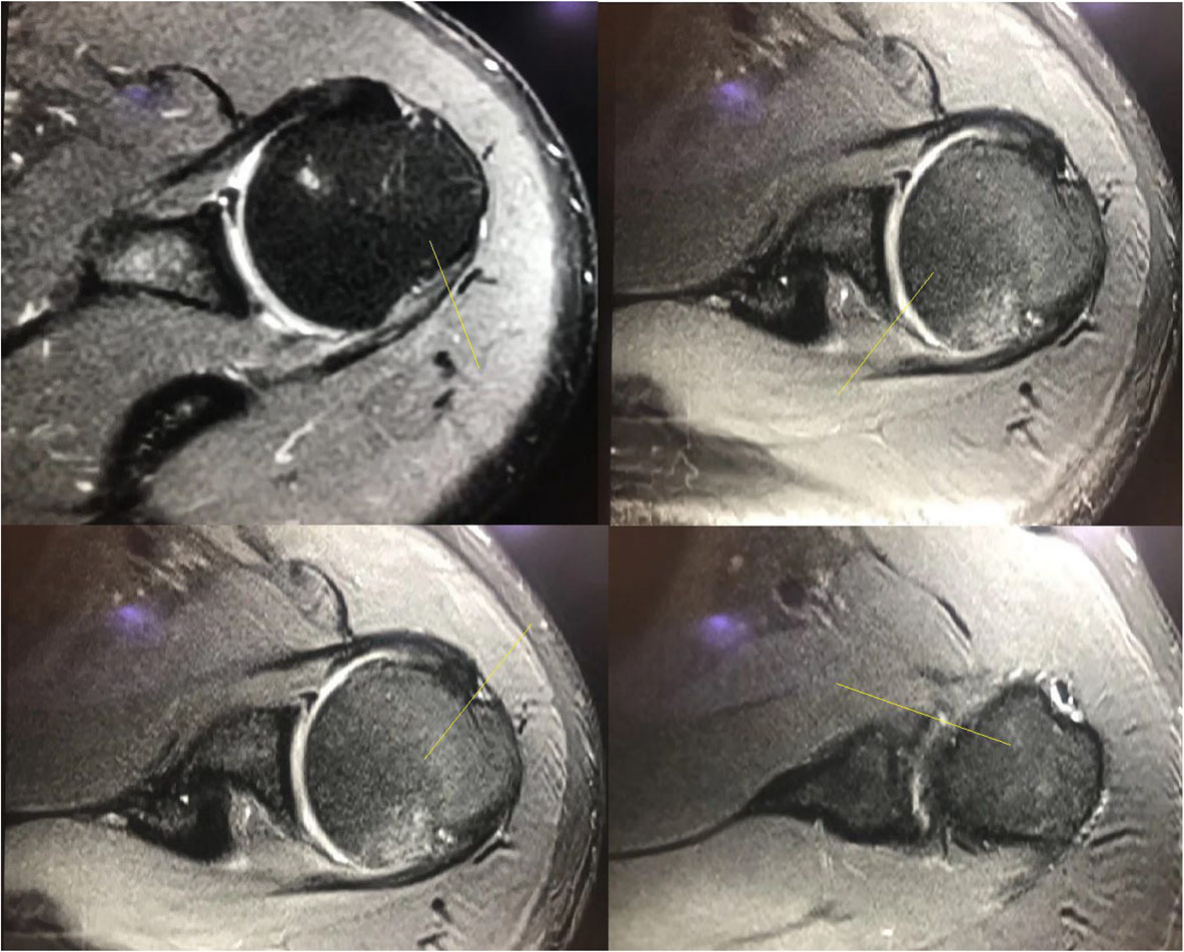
Postsurgical radiographic images of 29-years female patient with shoulder instability showed the union of the grafts during follow-up. Supine view. The yellow line indicates the union of the Bankart lesions
